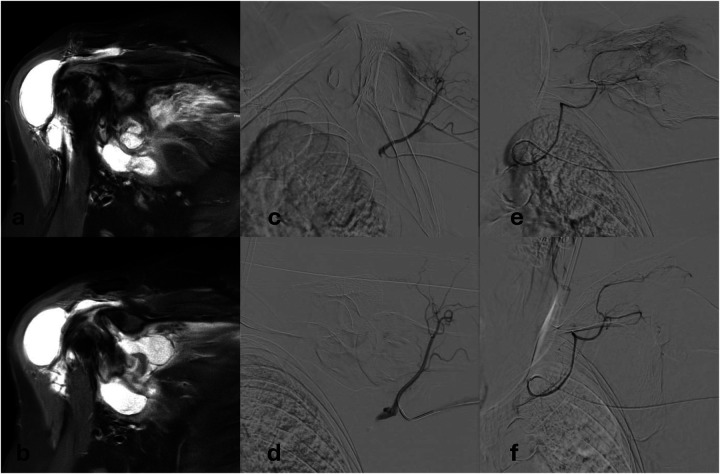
MRI before the procedure shows a 54-year-old man affected by a condition of distension and inflammation of the capsular-ligamentous complex of the shoulder, extending to the superior, anterior, and inferior recesses, in addition to bursitis of the sub-acromial deltoid, sub-coracoid, and subscapular bursae (a,b). Angiographic series of shoulder images before and after TAE (c–f). Selective angiography of pathologically inflamed arteries before embolization, characterized by contrast medium blush of the soft tissue (c,e). After embolization, the distal blush is no longer visible (d,f).
